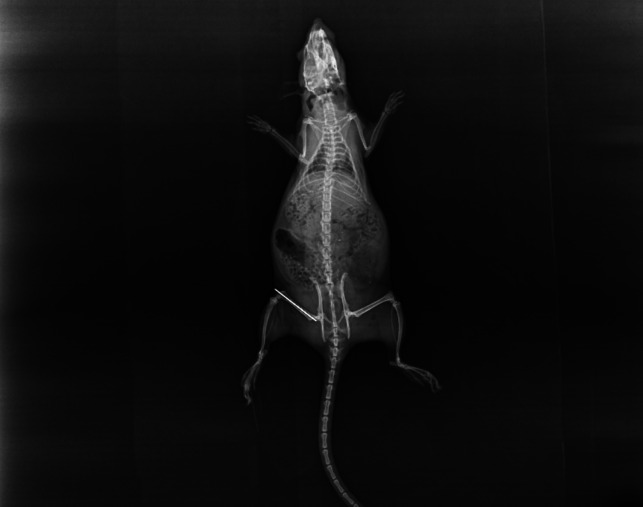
The control radiograph on postoperative day 0
